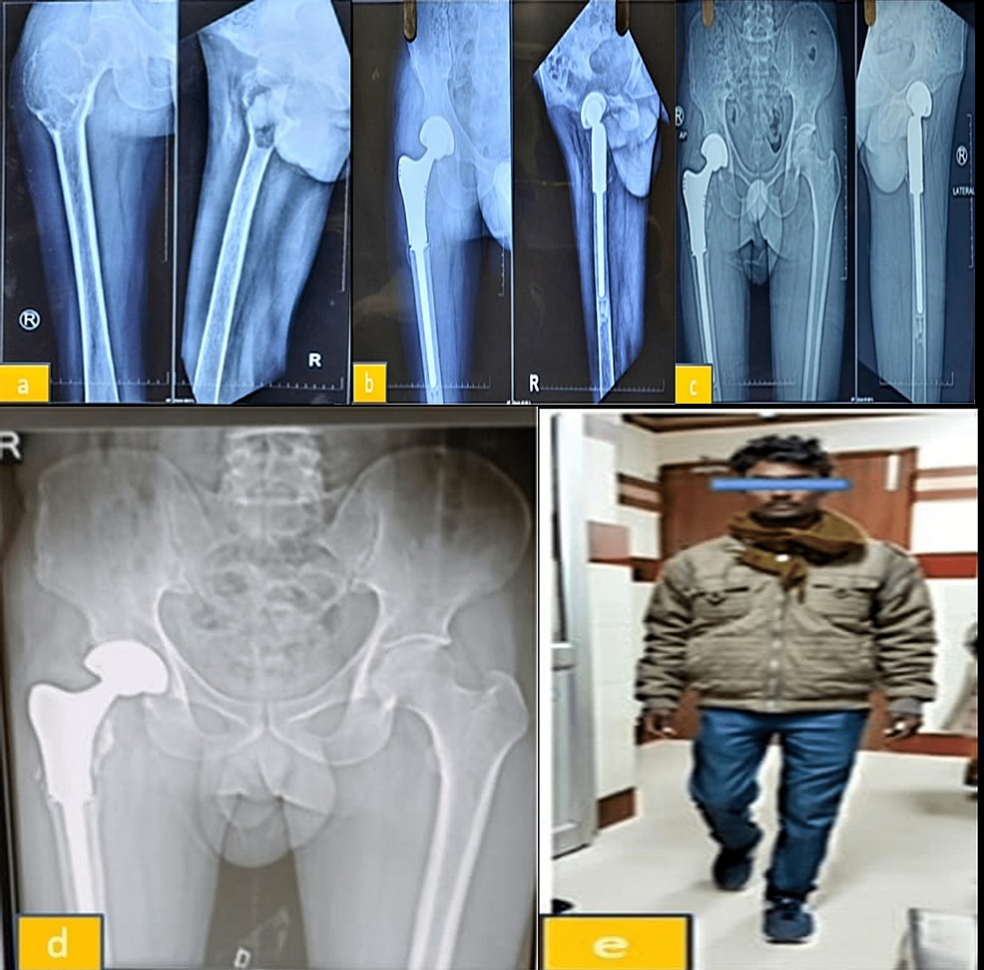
The bone growth around the mega prosthesis. (a) X-ray of the right proximal femur GCT (Enneking's stage IIA). (b) X-ray of the right proximal femur at three-month follow-up. (c) X-ray of the right proximal femur at 2.5-year follow-up. (d) X-ray of the right proximal femur at three-year follow-up showing new bone formation at the calcar of the endoprosthesis. (e) Patient photograph while walking at three-year follow-up. GCT: giant cell tumour.
